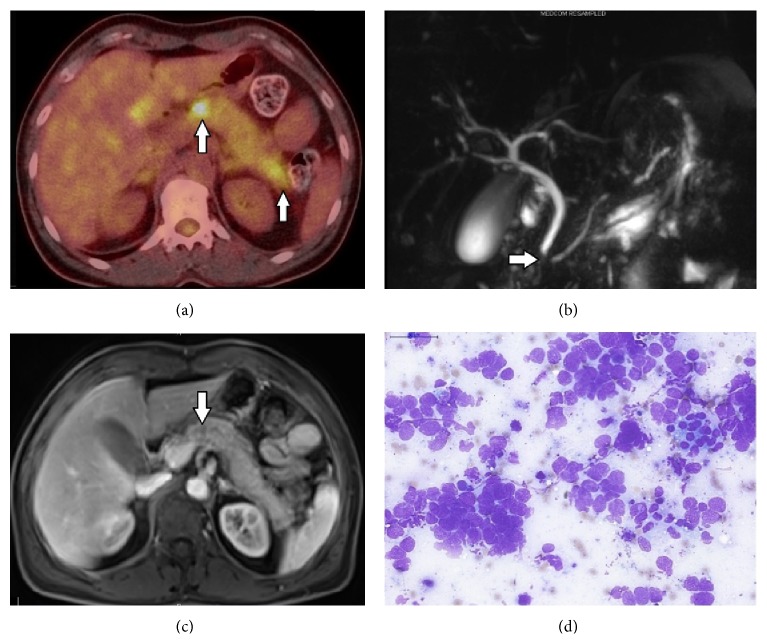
(a) Focal involvement areas of neck and tail of the pancreas on PET-CT (indicated by arrows). (b) Indistinct and nonuniformly circumscribed area of the pancreatic neck on abdominal MRI (arrow). (c) Segmental obliteration of the pancreatic duct on MRCP (arrow) and dilatation to its distal part. (d) Small cell carcinoma as shown by cytopathological examination of EUS-FNA taken from suspected area of the pancreas (MGG, ×40).
